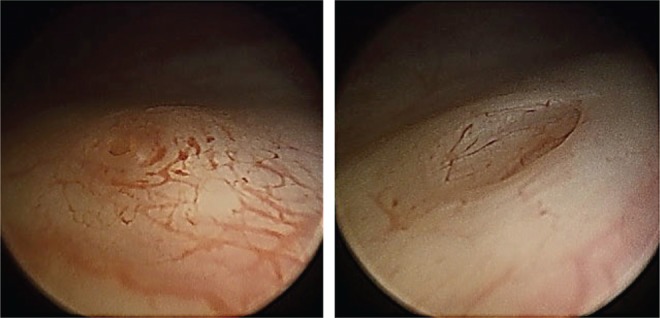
Different looking right and left ureteral orifices in the same individual. CT urogram and retrograde studies have proven normal orifices.
